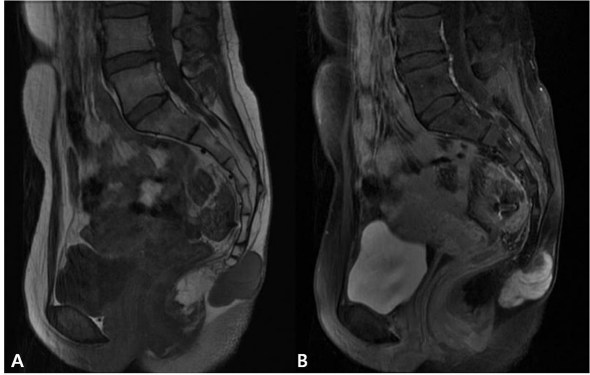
MR image shows the well circumscribed mass near the coccyx. The tumor was lobulated with low signal T1-weighted (A) and high signal T2-weighted image (B).
